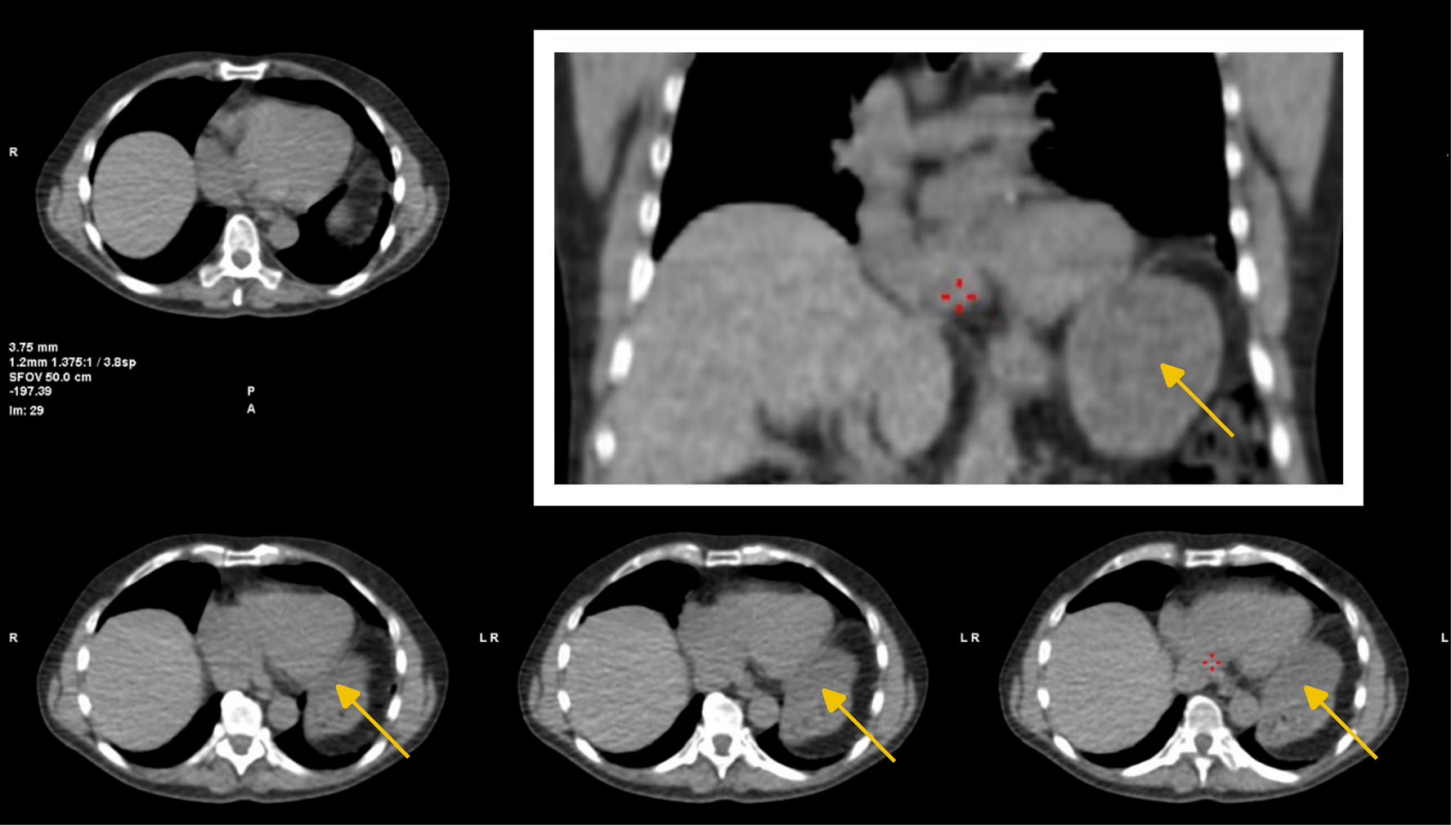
Low-dose computed tomography images (upper left and lower images: transversal slices; upper right image: coronal slice) used for attenuation correction showing an elevated diaphragm, which on the left side resulted in intestinal tissue (yellow arrows) being located in close proximity to the inferior and lateral left ventricular walls. This resulted in attenuation of normally perfused inferior and lateral walls and, in turn, regions with true defects to falsely appear relatively normal
